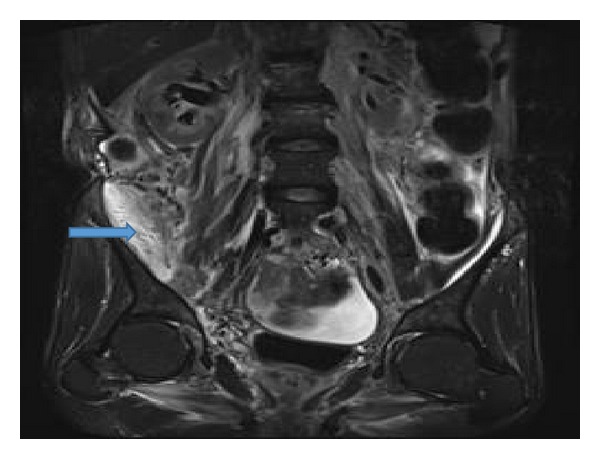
T2 coronal MR imaging showed enlarged predominantly hyperintense right psoas and iliopsoas (as pointed by arrow) without any discrete fluid collection. Right kidney displaced anteriorly and laterally.
